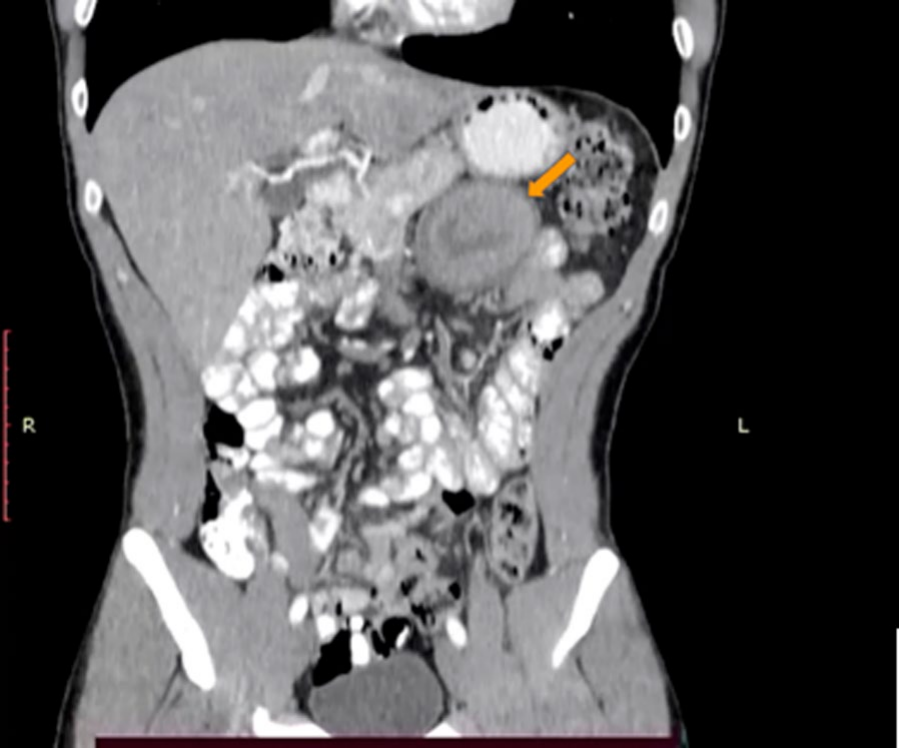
Coronal section of abdominopelvic computed tomography with double contrast, lesion marked with an orange arrow
